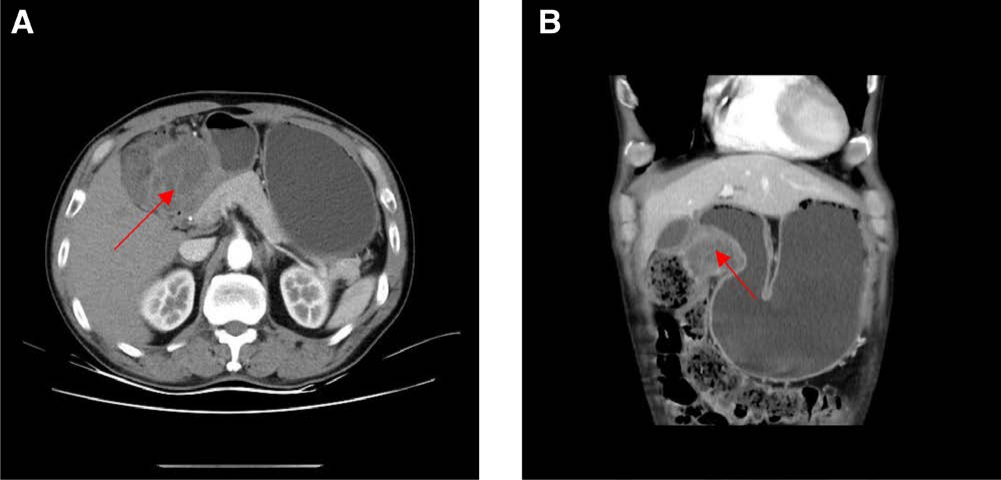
Contrast-enhanced CT scan on admission showed a mass measuring 5.2 cm in maximum diameter located among gastric antrum, pancreas, and transverse colon, and involved part of transverse colon and head of pancreas (arrow). (A) Transverse section; (B) coronal position.
